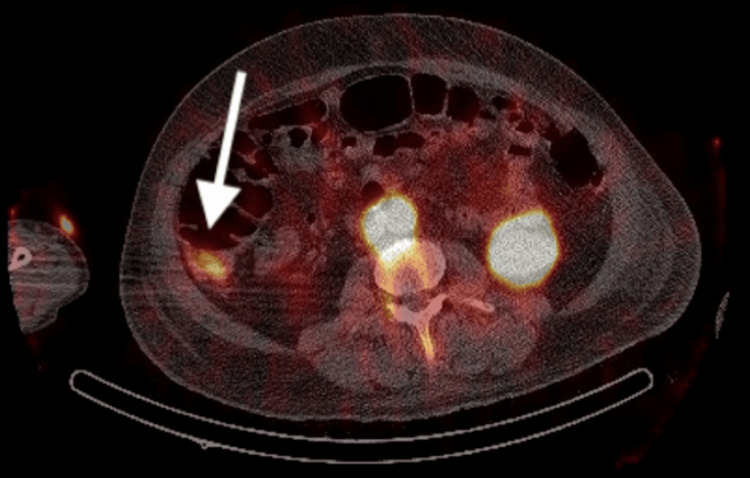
Axial section of a Technetium-99m labelled red blood cell SPECT-CT study demonstrating radionucleide accumulation within the ascending colon
